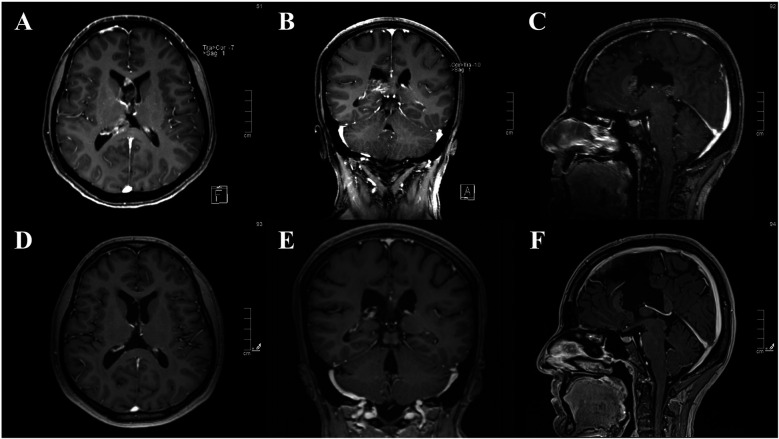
(A–C) contrast-enhanced MRI images of local recurrent central neurocytoma in the right lateral ventricle. (D–F) Post-RT contrast-enhanced MRI images of complete regression.
